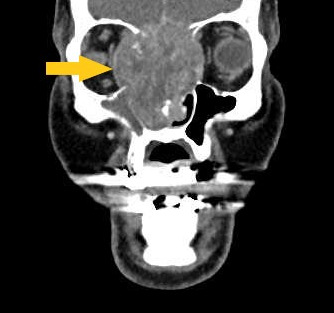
Preoperative multi-slice computed tomography (CT). The arrow points to the tumor.
